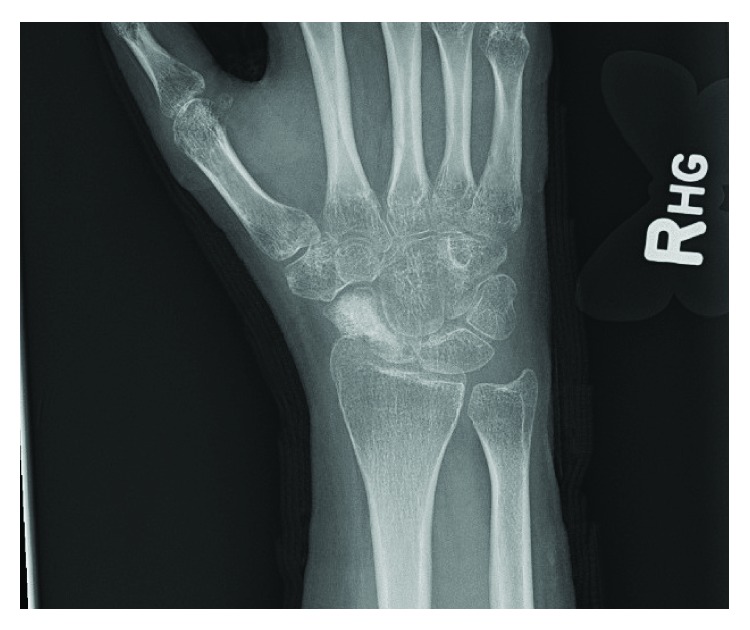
AP radiograph of the right wrist, demonstrating early consolidation of the autograft without evidence of local recurrence. 4-month follow-up X-ray.
